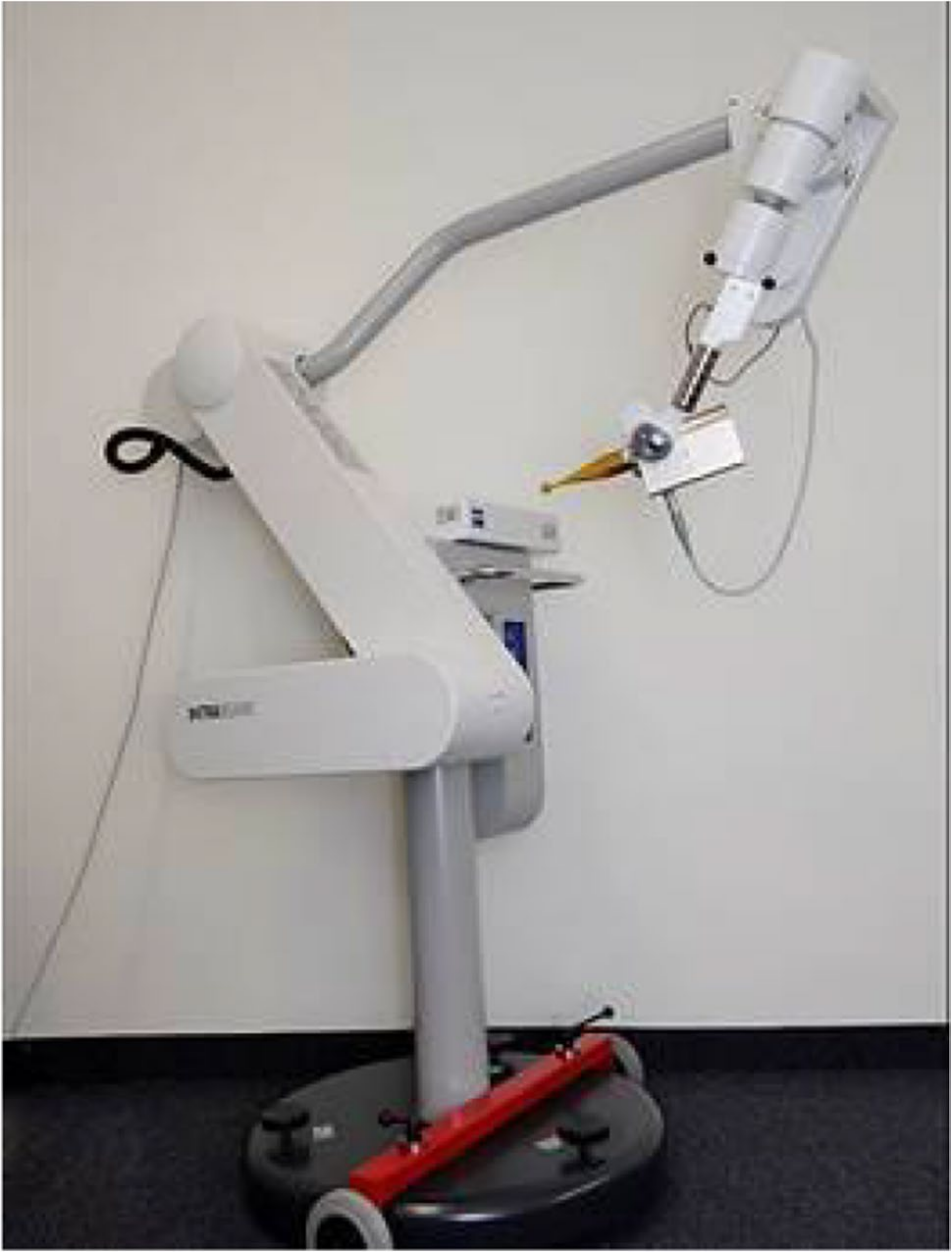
After inserting the applicator sphere, intraoperative irradiation is performed with 50-kV x-rays via an INTRABEAM system
